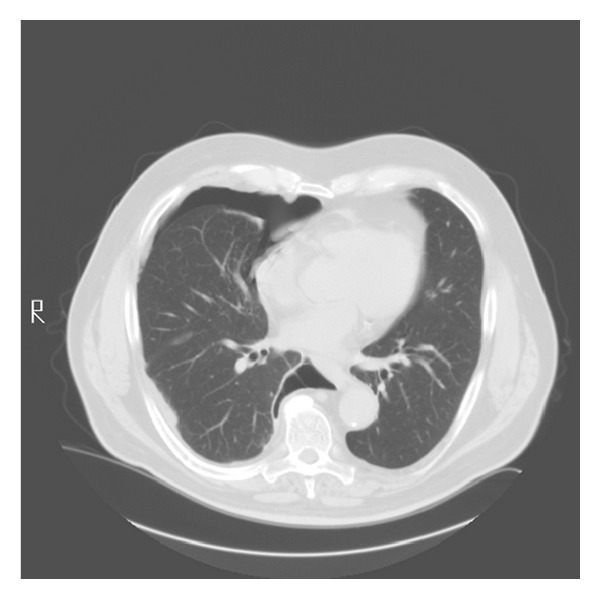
Chest CT scan in March 2008.
